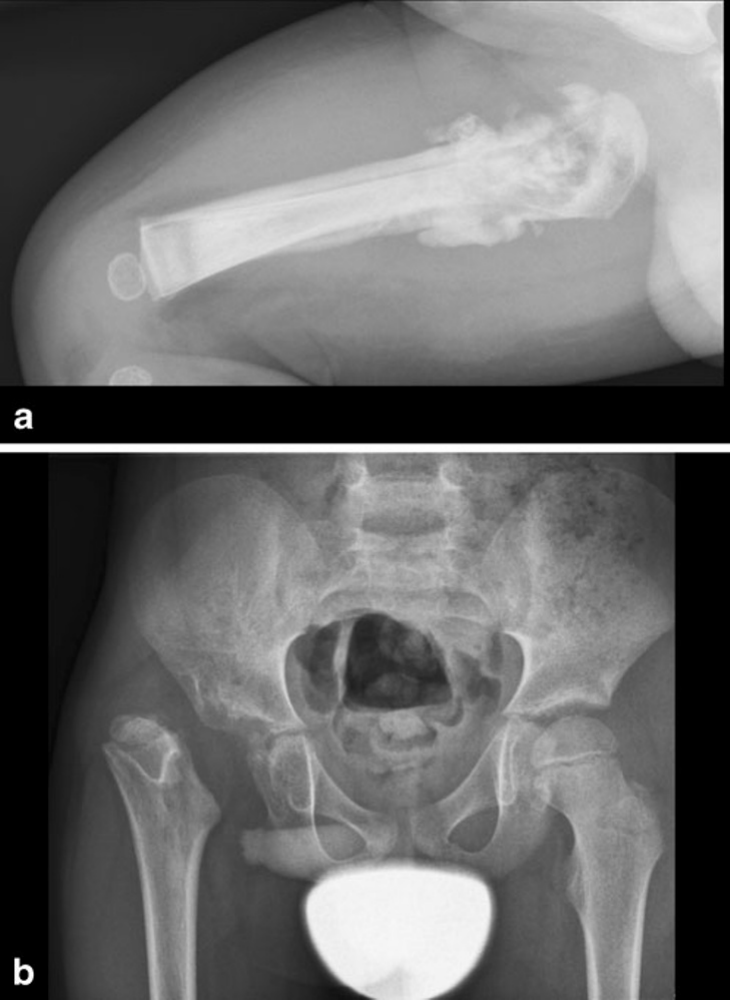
a A 6-week-old boy with fever. X-ray shows extensive cortical destruction of the proximal metaphysis with surrounding soft tissue calcifications and periostal reaction. This was proven to be septic osteoarthritis. b At age 7 years X-ray shows (acquired) deformity of the caput femoris, with luxation
